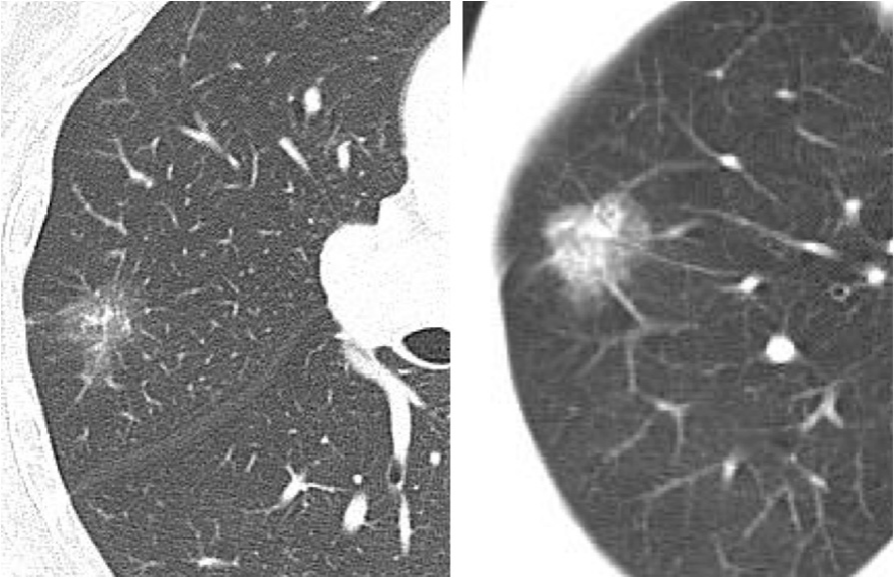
CT findings of pure (left) and mixed (right) ground-glass opacity lesions.
